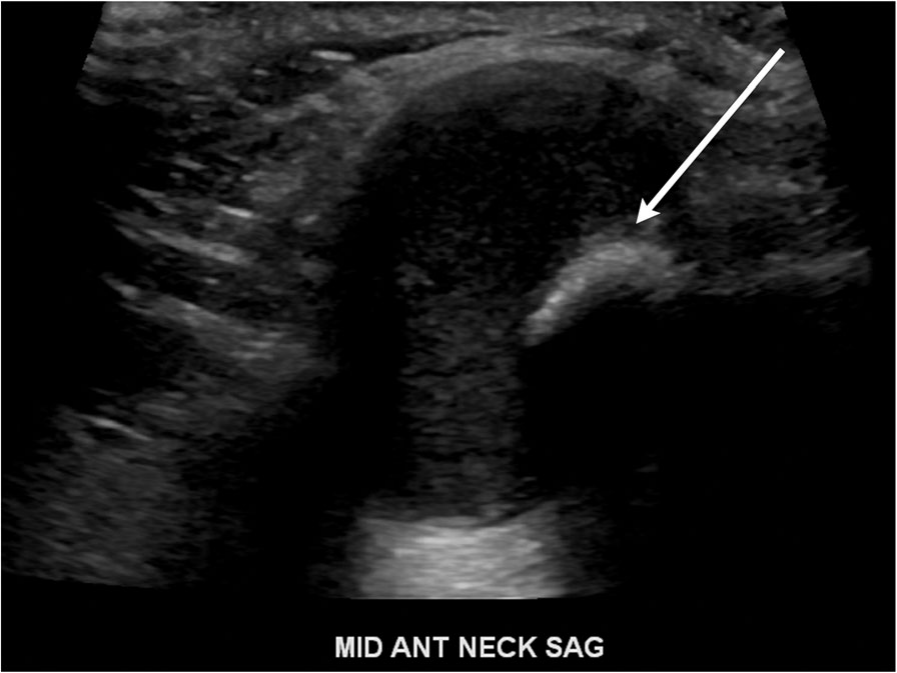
Infrahyoid thyroglossal duct cyst. Long axis grayscale ultrasound image shows a midline cyst in contact with and extending posterior to the hyoid bone (arrow)
